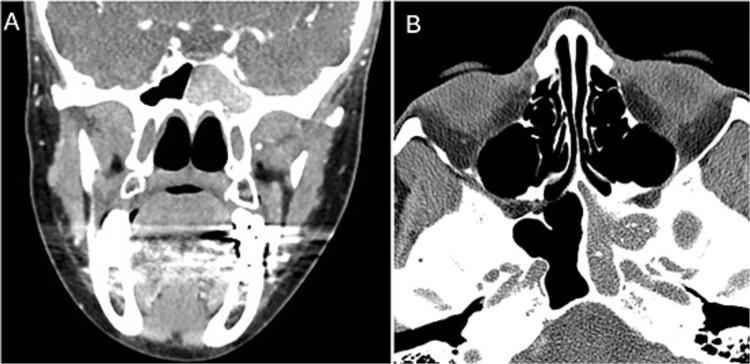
Coronal (A) and axial (B) images of a nonenhanced CT scan of the paranasal sinuses showing left sphenoid sinus complete homogenous opacification.
